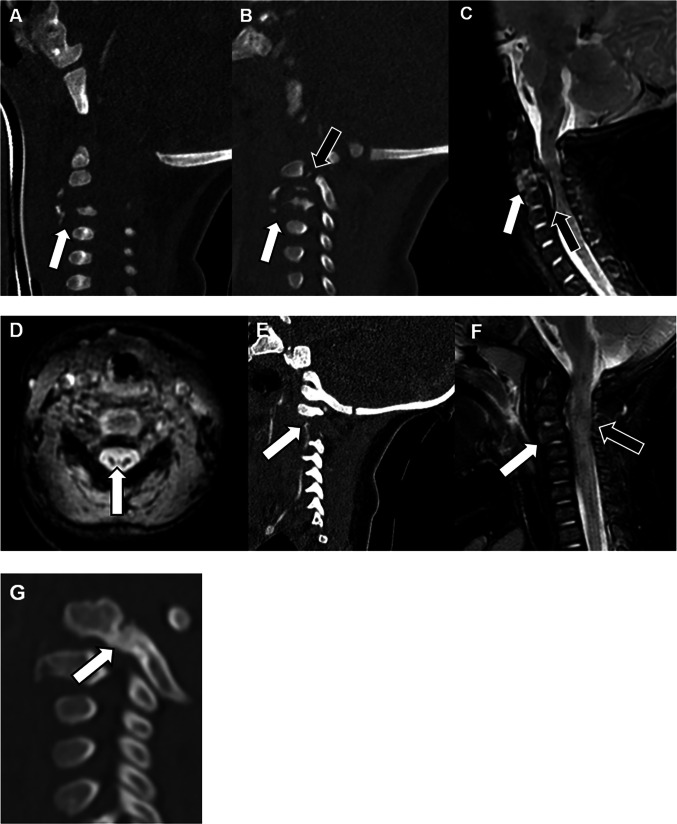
A 2-month-old female with severe abusive head and spine trauma, with follow-up cervical spine imaging at 4 months and 7 months of age. Sagittal CT images in midline (A) and off-midline (B) demonstrate extensively comminuted C3 burst (*white arrows*) and displaced C2 pedicle (*black arrow*) fractures, with anterolisthesis of C2 on C3. The C2 pedicle fractures were bilateral (contralateral fracture not pictured). Sagittal STIR MRI (C) shows edema associated with the burst fracture (*white arrow*) and uplifting of the posterior longitudinal ligament (*black arrow*) leading to spinal cord compression. Axial T2* MRI (D) demonstrates blooming artifact in the spinal cord due to intramedullary hemorrhage. Sagittal CT angiography maximum intensity projection image of the neck (E) shows left vertebral artery dissection (*arrow*). Right vertebral artery dissection was also present (not shown). Sagittal T2-weighted mDixon water-only image (F) obtained at 4 months of age, following non-operative treatment with immobilization, demonstrates resolution of acute findings including C3 vertebral body edema (*whitearrow*) and posterior longitudinal ligament injury, as well as mildly increased caliber and T2-hyperintense signal of the spinal cord (*blackarrow*). Sagittal CT image (G) at 7 months of age demonstrates partial healing of the C2 pedicle fracture (*arrow*)
